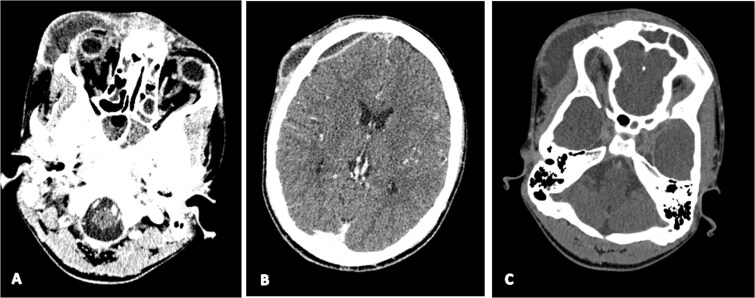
Axial contrast-enhanced brain CT showing right orbital cellulitis (A), right frontal empyema (B), and bilateral cavernous sinus thrombosis (C).
